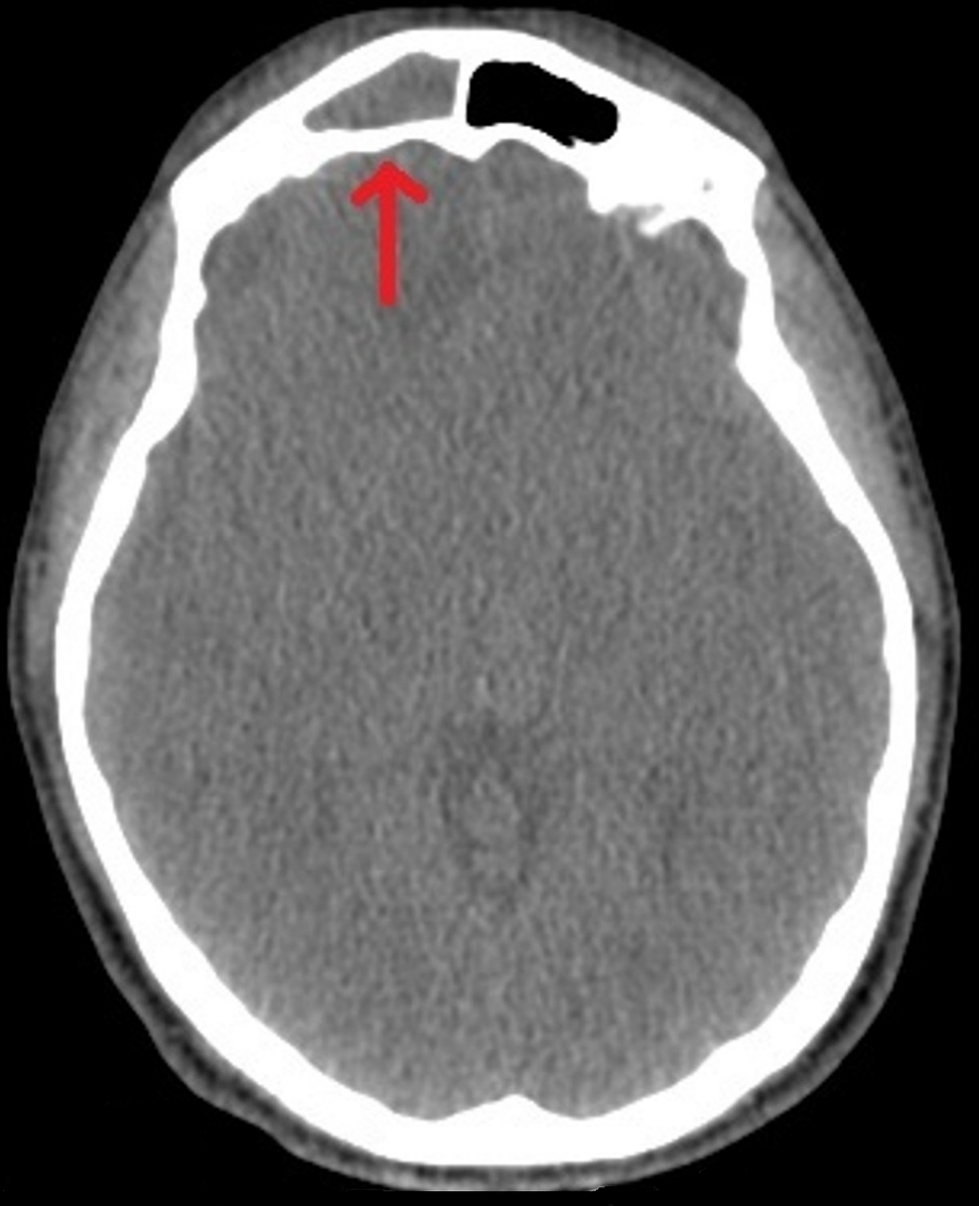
CT showing opacified right frontal sinus.
